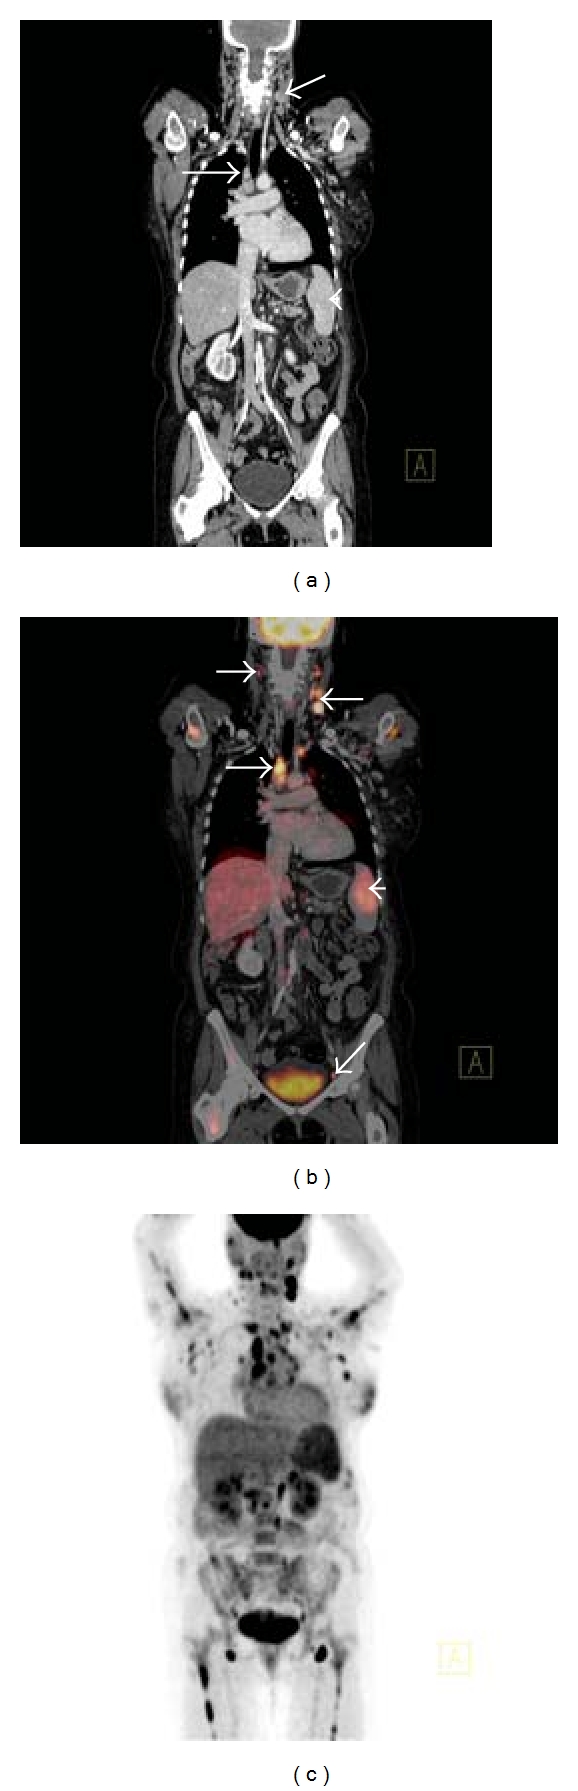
Coronal CT (a) demonstrating left cervical and right paratracheal lymphadenopathy (white arrows) and mild splenomegaly (white arrowhead) consistent with high-grade follicular lymphoma. Corresponding coronal fused FDG-PET/CT (b) demonstrates increased radiotracer uptake in the pathologically enlarged left cervical and right paratracheal lymphadenopathy (white arrows) and spleen (white arrowhead), but also abnormal FDG accumulation in right cervical and left external iliac lymph nodes (white arrows) that appeared normal by size criteria on the corresponding CT scan. Coronal PET MIP (c) in the same patient demonstrating widespread increased radiotracer uptake throughout the neck, thorax, abdomen, and proximal humeri and femora bilaterally; the widespread bone marrow involvement was not apparent on CT.
